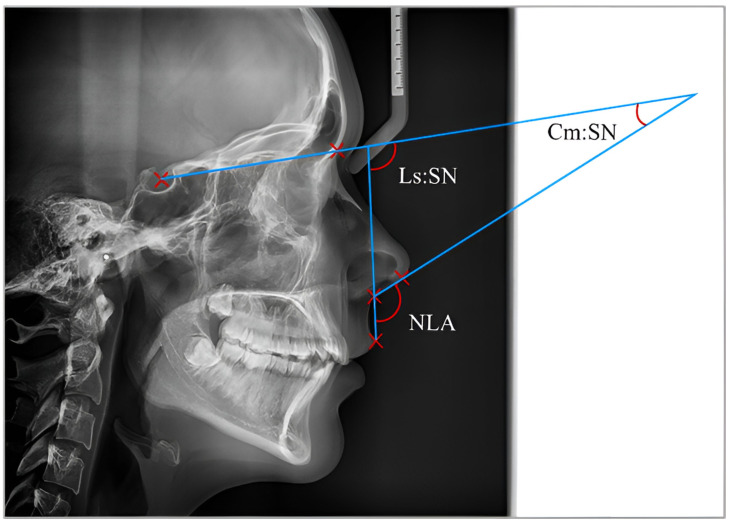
Nasolabial angle measurements on the lateral radiograph: the tangent of the base of the nose to the SN plane and the tangent of the upper lip to the SN formed two angles, Cm:SN (columella:sella–nasion angle) and Ls:SN (labrale superior:sella–nasion).
